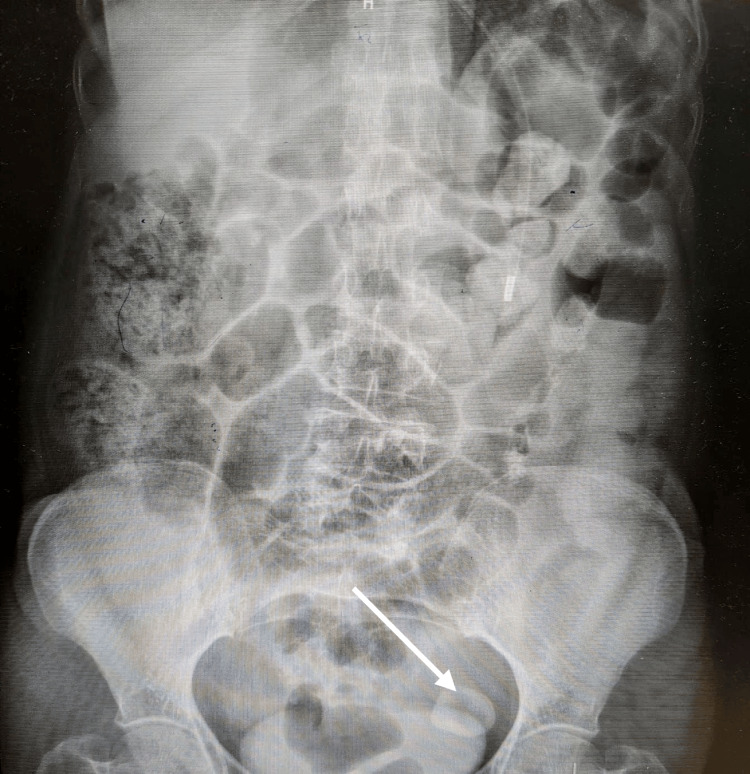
Plain abdominal radiograph demonstrating dilated small bowel loops with a radio-opaque intraluminal calculus (arrow), suggestive of an enterolith.
